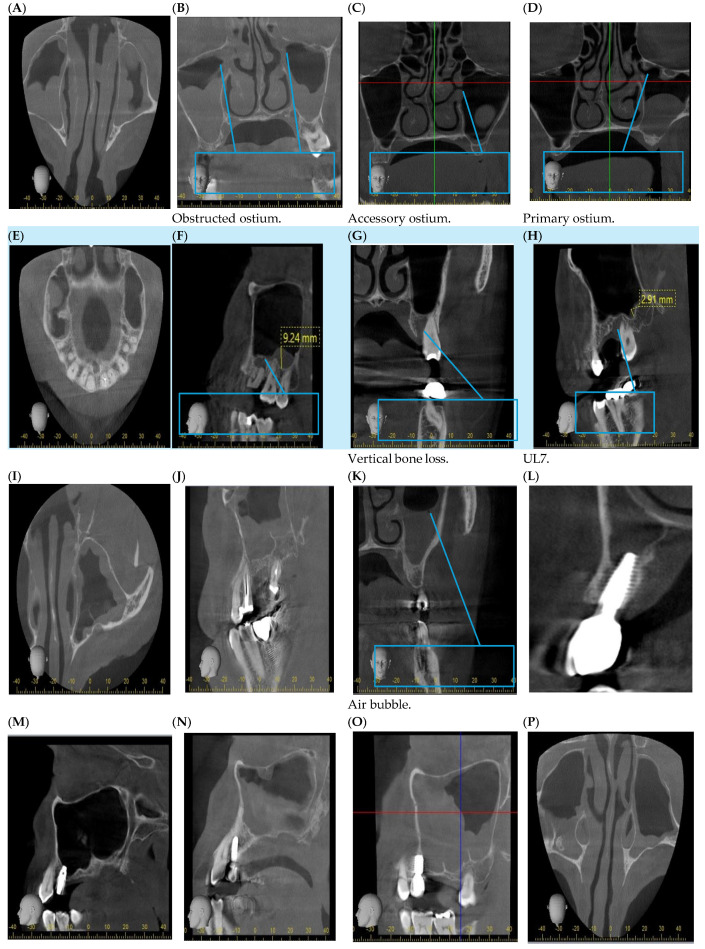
Representative CBCT images of incidental findings in the maxillary sinus. (A,B) Bilateral polypoid, circumferential mucosal thickening >10 mm with ostium obstruction. (C,D) Accessory ostium with polypoid thickening >10 mm and patent primary and accessory ostia. (E,F) Apical pathology (UR6) associated with unilateral polypoid thickening (5–10 mm). (G,H) Periodontal bone loss (UL7) with flat mucosal thickening (2–5 mm). (I,J) Periodontal disease and root-filled teeth associated with circumferential polypoid thickening >10 mm. (K,L) Air–fluid level associated with implant breaching the sinus floor (UL4). (M) Implant breaching sinus floor without mucosal thickening. (N,O) Bilateral implants (UR5, UL4) with circumferential polypoid thickening >10 mm and ostium obstruction. (P) Antrolith within the right maxillary sinus. Abbreviations: UR = upper right; UL = upper left.
